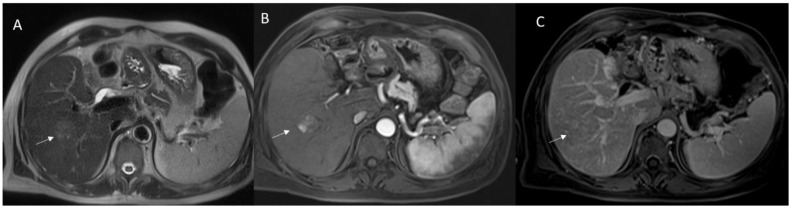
MRI of HCV 47-year-old patient with a case of intrahepatic cholangiocarcinoma. In T2-weigthed sequence (A), the lesion (arrow) shows inhomogeneous signal, iso-hyperintense. During vascular assessment ((B): arterial phase; (C): portal phase), the lesion is characterized as LR-M (rim APHE (in (B), white arrow) and without washout (in (C), white arrow)). (B,C): gradient T1-weigthed sequences (Volumetric interpolated breath-holding examination sequence), in axial plane in arterial and portal phases.
